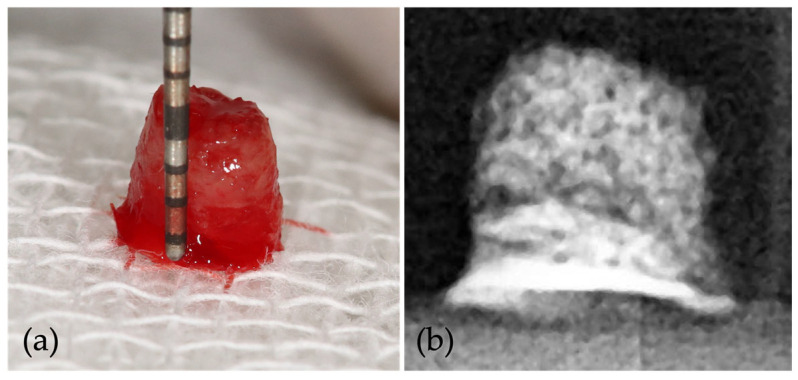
X-Ray image of the implanted ABBM after eight weeks. (a) Gross observation. (b) X-Ray image of the implanted ABBM.
